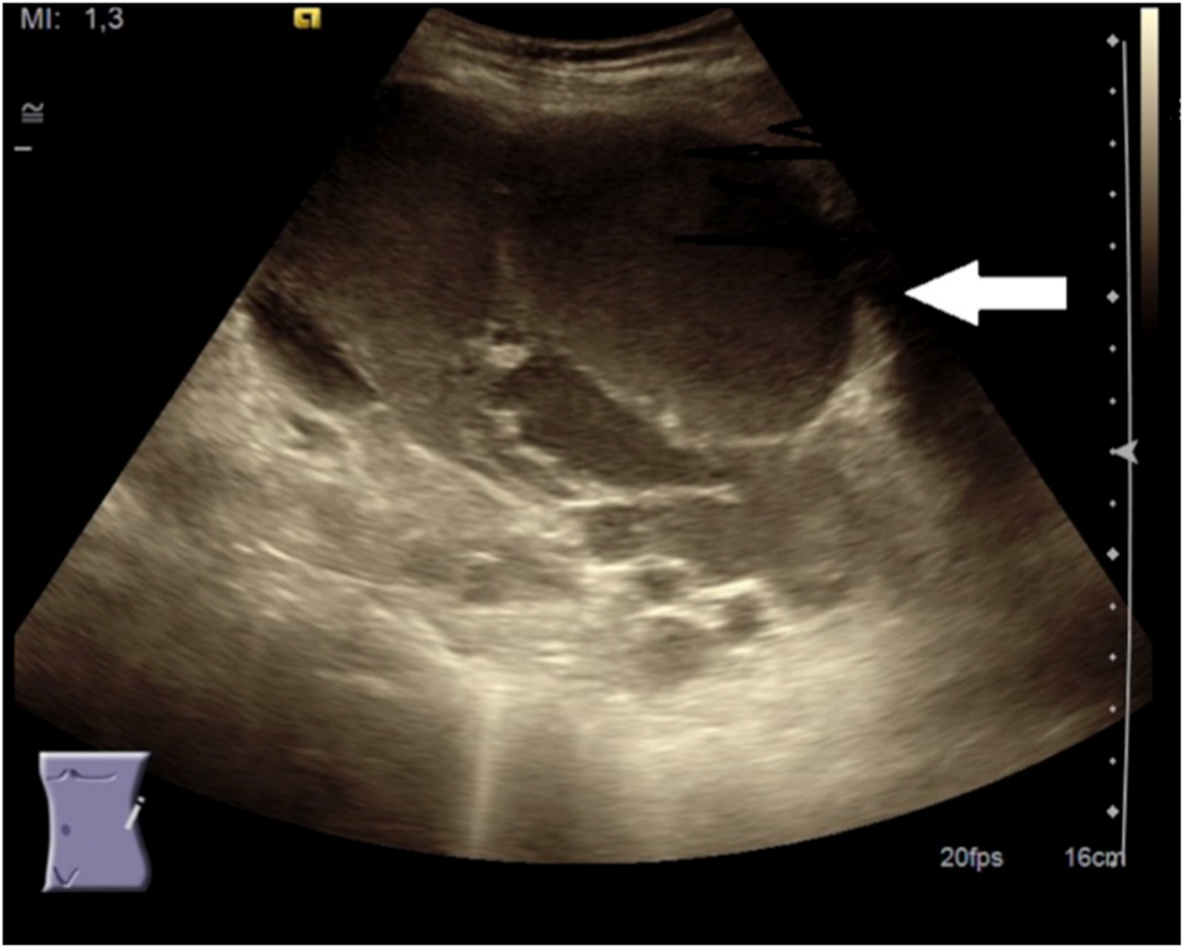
Ultrasound showed a large cystic lesion with septa and some sedimentation. Minimal Doppler signal of the large tumor (18 × 10 cm). Spleen, pancreas and left kidney without focal lesions
